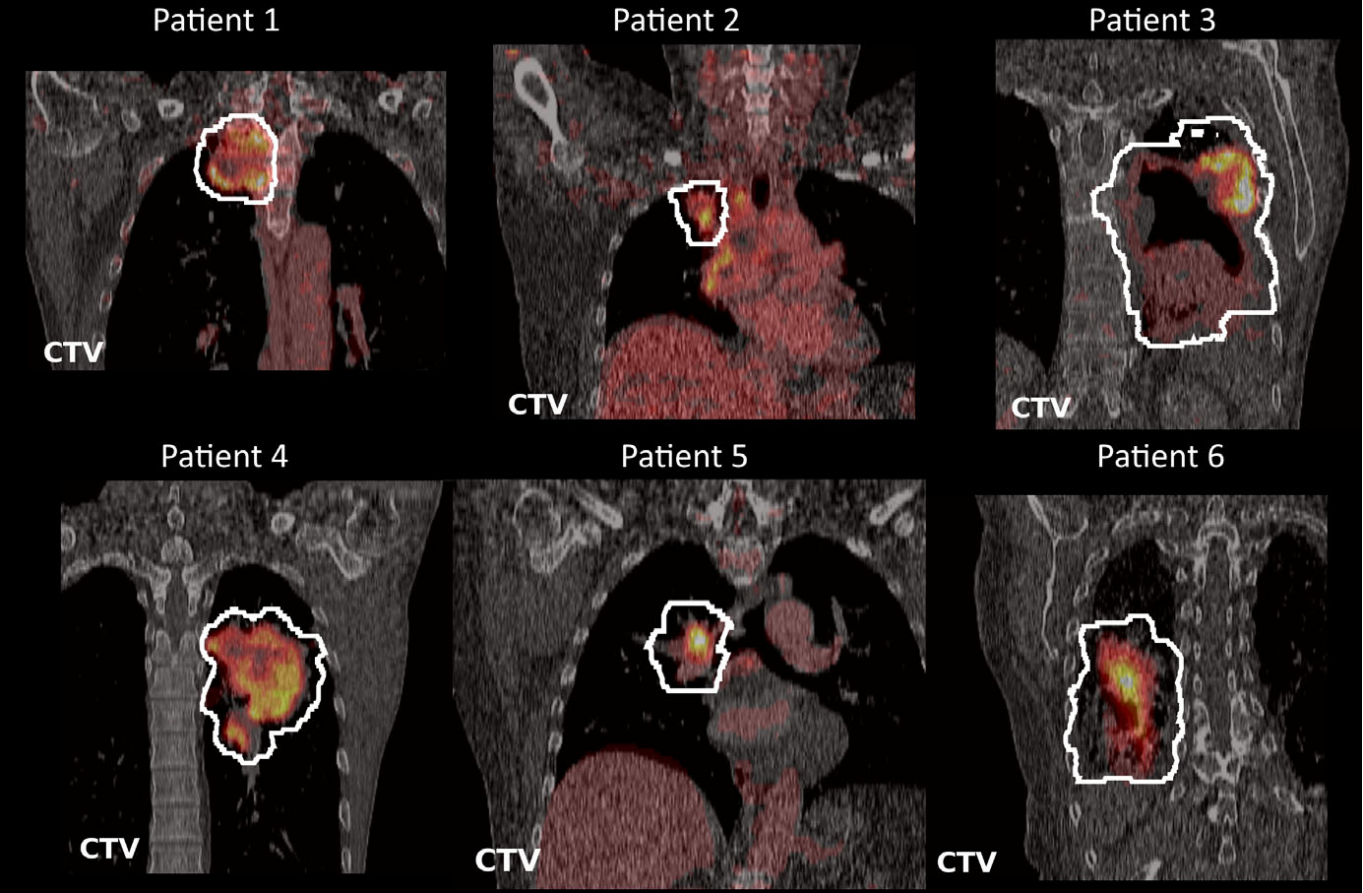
Coronal images of the of the FDG‐PETCT scans of the first six patients through the center of the CTV.
